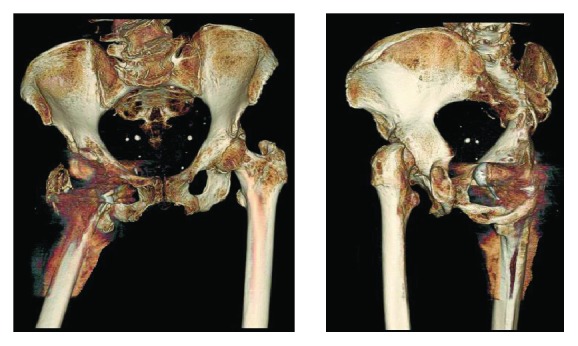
Three-dimensional reformatted computed tomography (CT) images showing femoral component dislocation through the right obturator canal.
